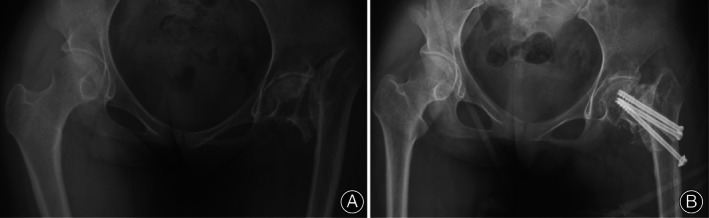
Radiographs of the patient's pelvis during this admission reveal a Garden IV femoral neck, old fractures are not union (A) and Postoperative review of the pelvis show left femoral neck fracture is well reduced and the fibula graft is in good position (B).
